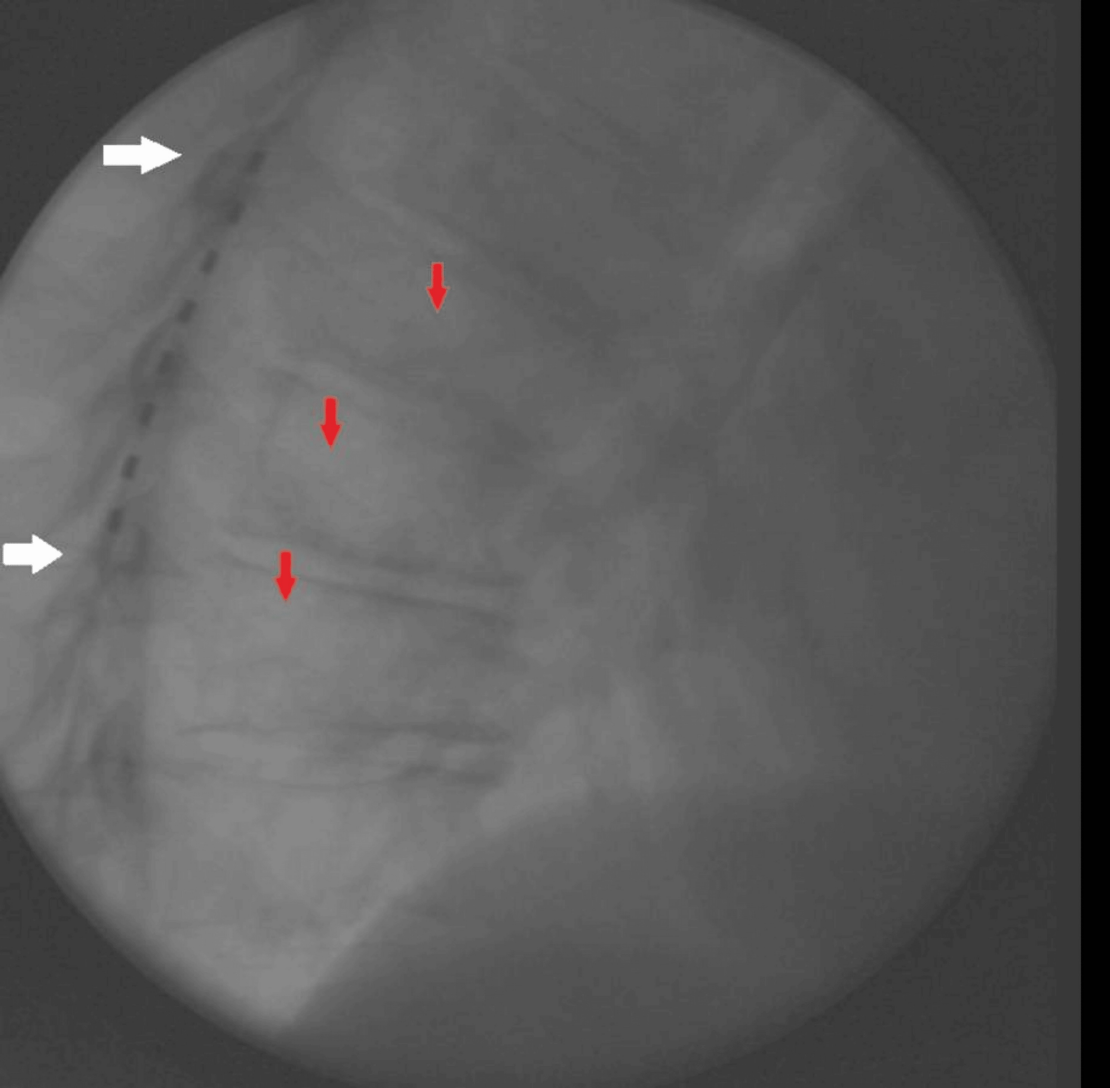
Lateral cervical fluoroscopic radiograph, post-implantation White arrows indicate the superior (at C7) and inferior edge of the SCS leads within the epidural space. Red arrows indicate posterior fusion screws. This image highlights the successful implantation of the SCS leads despite the presence of overlying surgical hardware.
